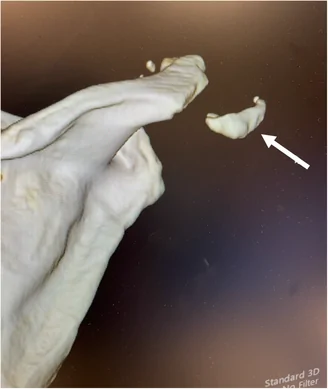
Preoperative 3D CT scan showing a right shoulder displaced lateral acromion fracture (white arrow) with a fracture line running in an anterior‐to‐posterior direction. This fracture fragment has a large portion of the anterior and middle deltoid attached to it.
